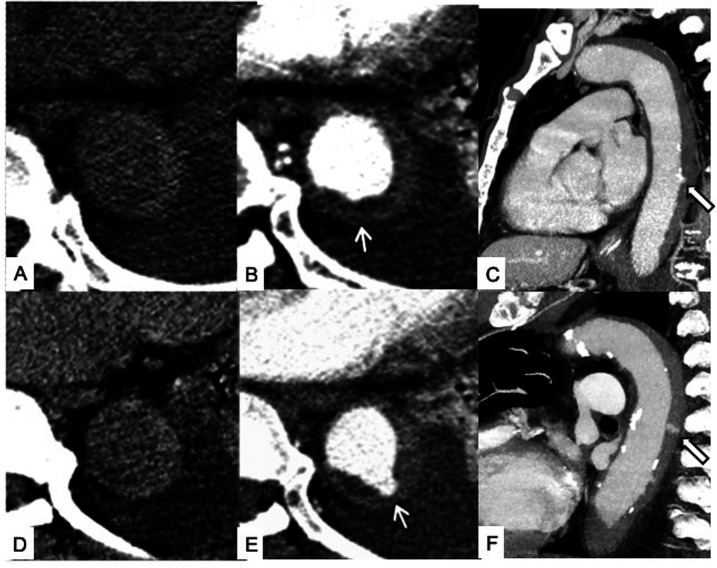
Temporal evolution of unstable Type B IMH. Initial unenhanced scan (A) and contrast-enhanced axial (B) and sagittal (C) CT images showing a Type B IMH with tiny intimal erosion of the descending aorta (white arrows). Four-day follow-up unenhanced scan (D) and contrast-enhanced axial (E) and sagittal (F) CT images at the same level showing enlargement of the intimal defect and enhancing ULP with neck >3 mm from the aortic lumen into the hematoma (white arrows), indicative of overt intimal tear and impending dissection.
